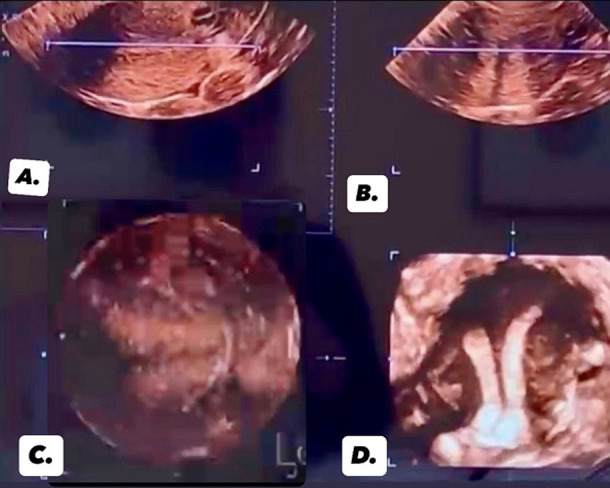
different ultrasound views for uterine didelphys: A) transverse view of uterus showing endometrial cavity in cross-section; B) sagittal view of uterus showing separation of uterine cavities; C) 3D coronal reconstruction of uterus; D) 3D coronal view of uterine cavity showing two distinct endometrial cavities, consistent with uterus didelphys
